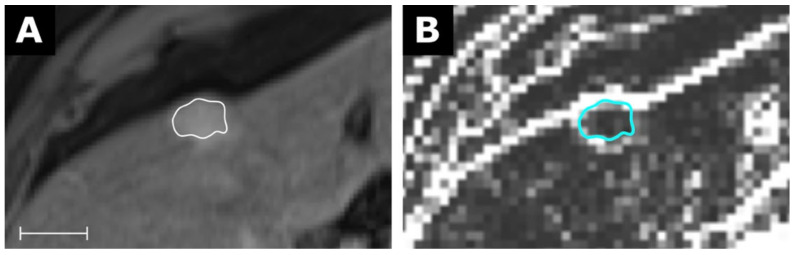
MRI of a hepatocellular carcinoma (A) with a corresponding map for the feature Variance with a resolution of 3 × 3 × 3 mm in (B). Scale indicates 2 cm. The value for Variance from the conventional extraction was 882 and 108 for the map. Details are available in electronic Table S4.
